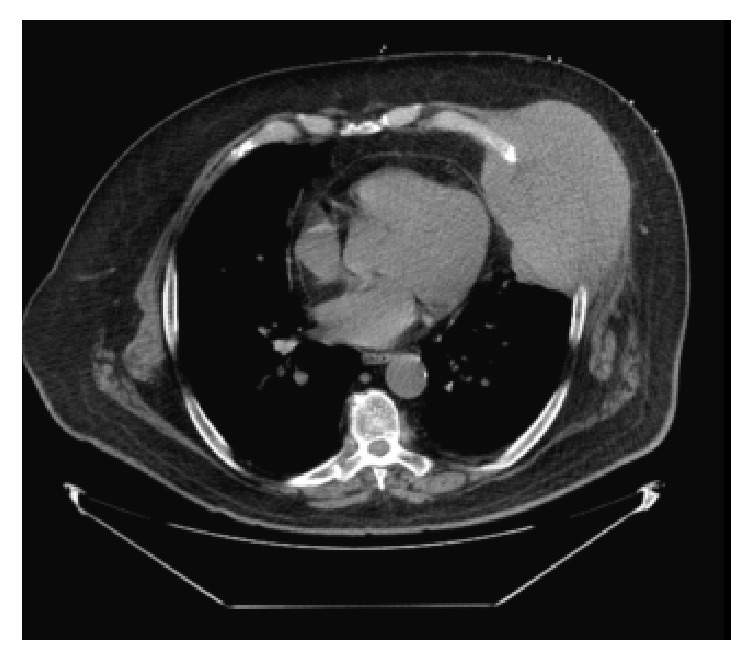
CT of chest with contrast showing 10 × 13 cm soft tissue mass in the left anterior lower hemithorax, from the left anterior 6th rib to the EO muscle and left pericardium, inseparable from LV myocardium.
